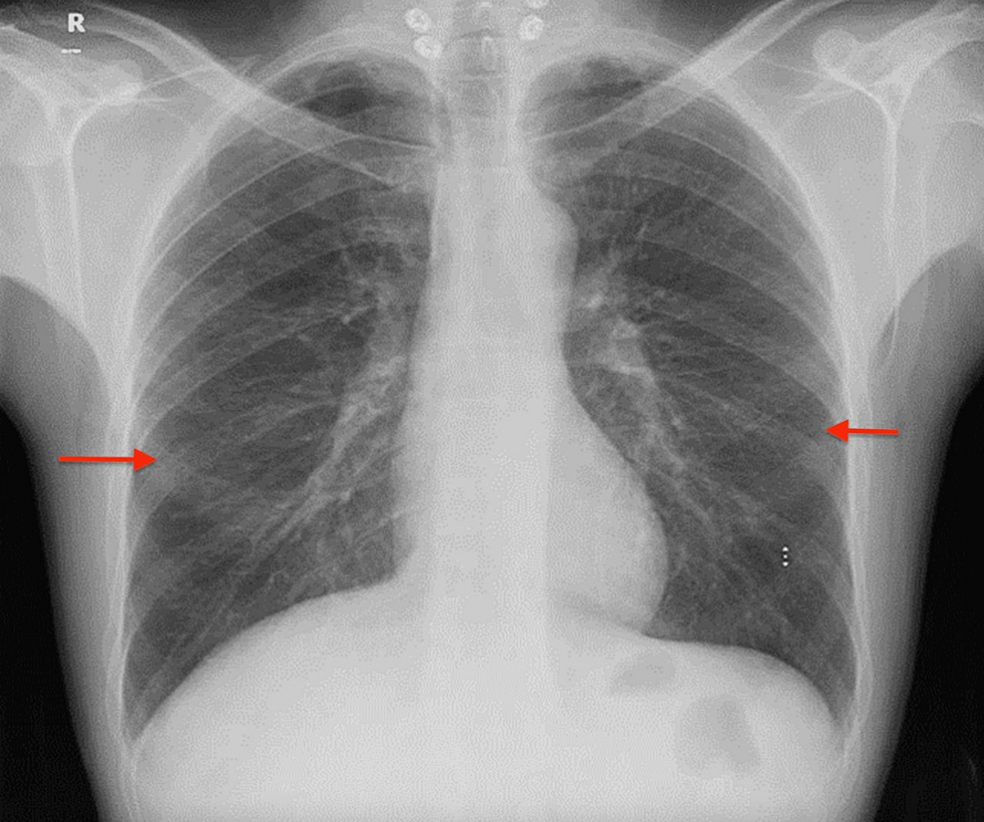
Chest X-ray on outpatient follow-up
